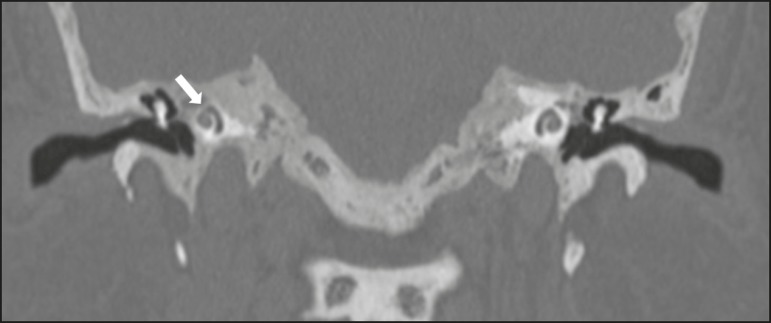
Paget's disease. Coronal CT scan, with bone window settings, showing
